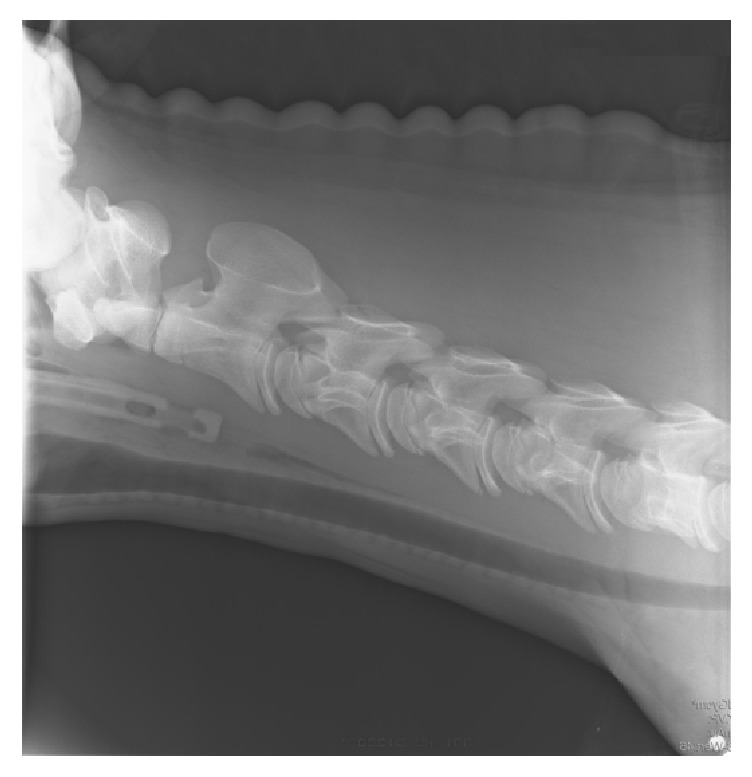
Lateral radiographic view of the cervical area. The nasogastric tube can be seen advanced up to the stricture site at approximately the second cervical vertebra.
